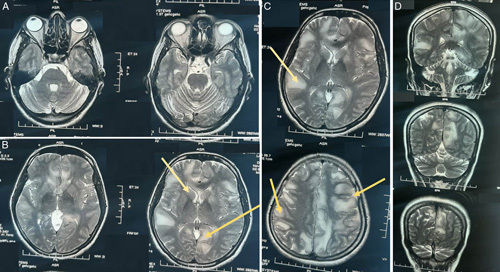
(A, B, C, D): T2-weighted MRI scan showing hyperintensities involving right temporal neocortex, bilateral symmetrical periventricular and subcortical white matter of frontal and parietal lobe.
